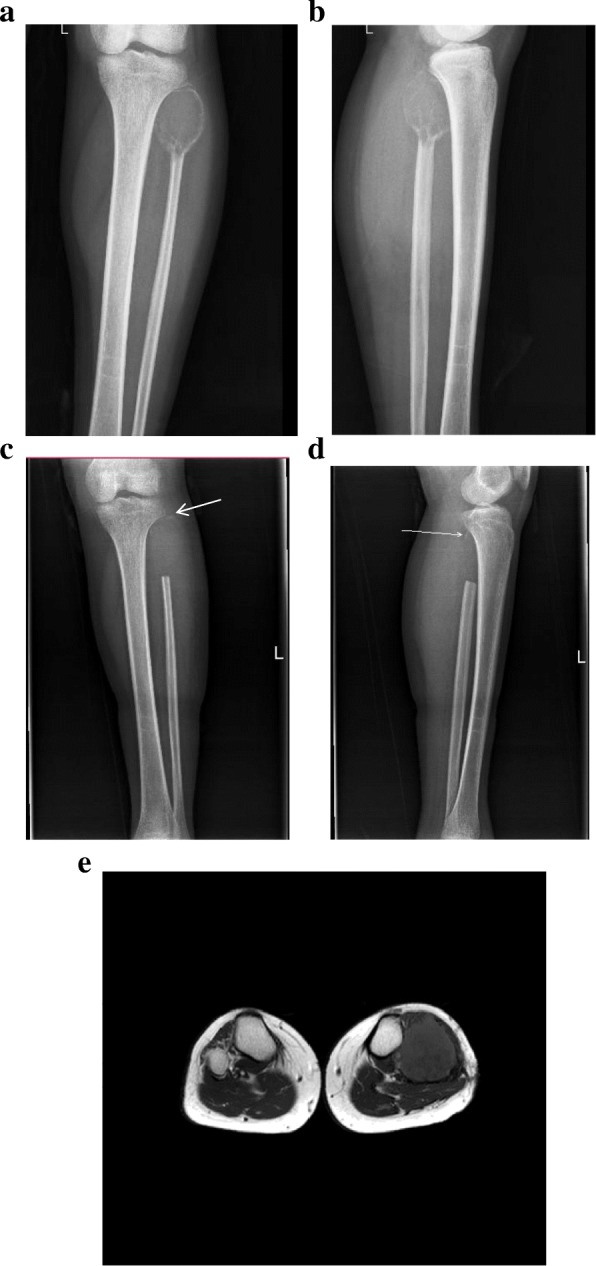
a, b Preoperative AP and lateral X-rays of the left leg of one of the patients showing the proximal fibular GCT. c, d Follow-up X-rays of the left leg of one of the patients, showing a residual bone fragment (arrows) 1 month postoperatively. e MRI of the same patient 8 months postoperatively, showing a soft tissue mass that had developed in the tibiofibular joint area
